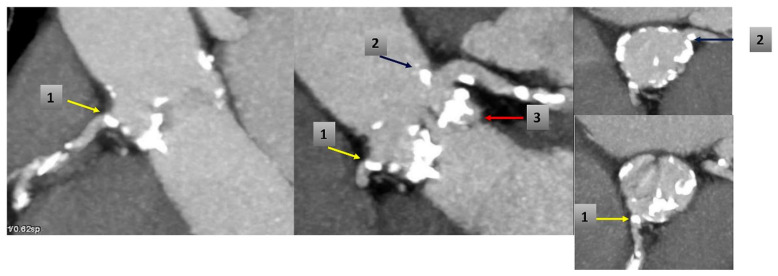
CT scan before TAVR shows: (1) RCA ostium calcification (yellow arrow); (2) calcification near ostium of the Left Main (blue arrow) and (3) calcified native valve under left coronary ostium (red arrow).
